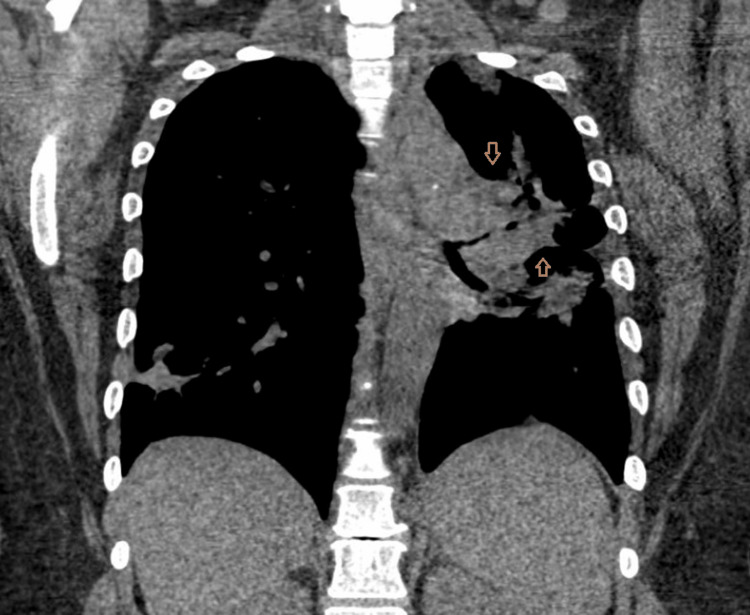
Coronal CT reconstruction demonstrating left-sided cavitary lesions with lung volume loss and multiple contralateral right-sided pulmonary nodules (arrows).
